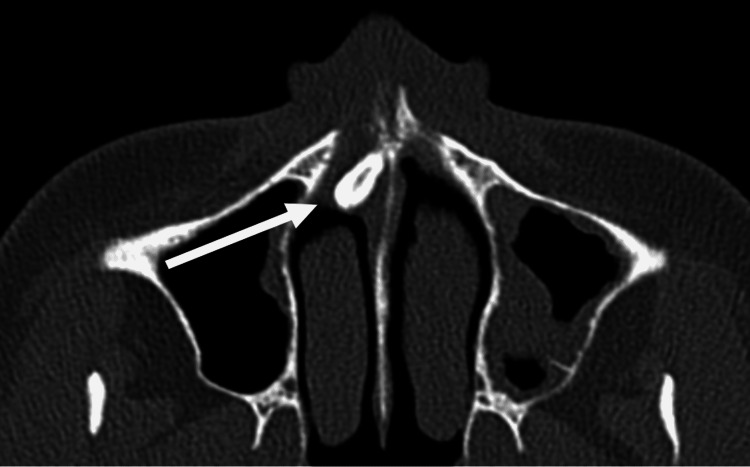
Preoperative axial computed tomography imaging of the paranasal sinuses showing an intranasal tooth (white arrow).
